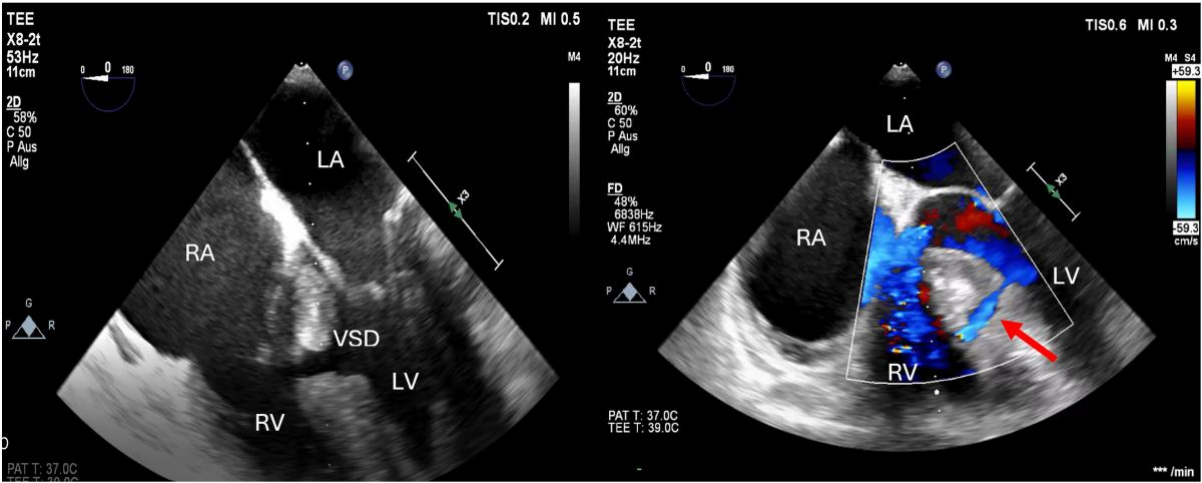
Transoesophageal echocardiography. Interventricular septal defect with left–right shunt (marked by red arrow); RA, right atrium; LA, left atrium; LV, left ventricle; RV, right ventricle; VSD, ventricular septal defect (©Medical University of Graz).
